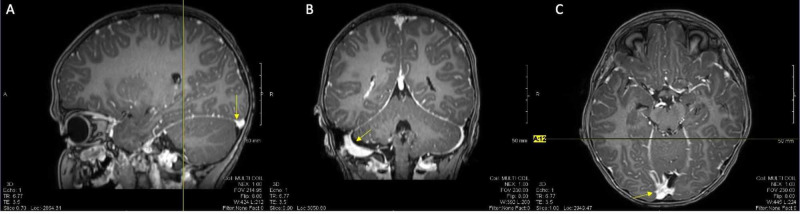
Initial brain MRI of patient 1 Evidence of hyperenhancement, portions identified with yellow arrows in the respective planes. (A) Sagittal view, (B) coronal view, (C) axial view.
